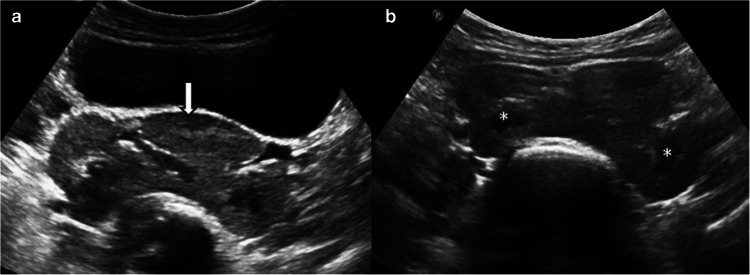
Pancake kidney. A 1-year-old girl presented to the hospital with recurrent urinary tract infections. Both kidneys were not visualized in the renal fossa. a Axial grayscale ultrasound (US) image shows a large, lobulated renal mass (*arrow*) posterior to the urinary bladder. b The medulla (*asterisk*) and cortex, exhibiting different echogenicity on US, are distinguishable, consistent with pancake kidney
